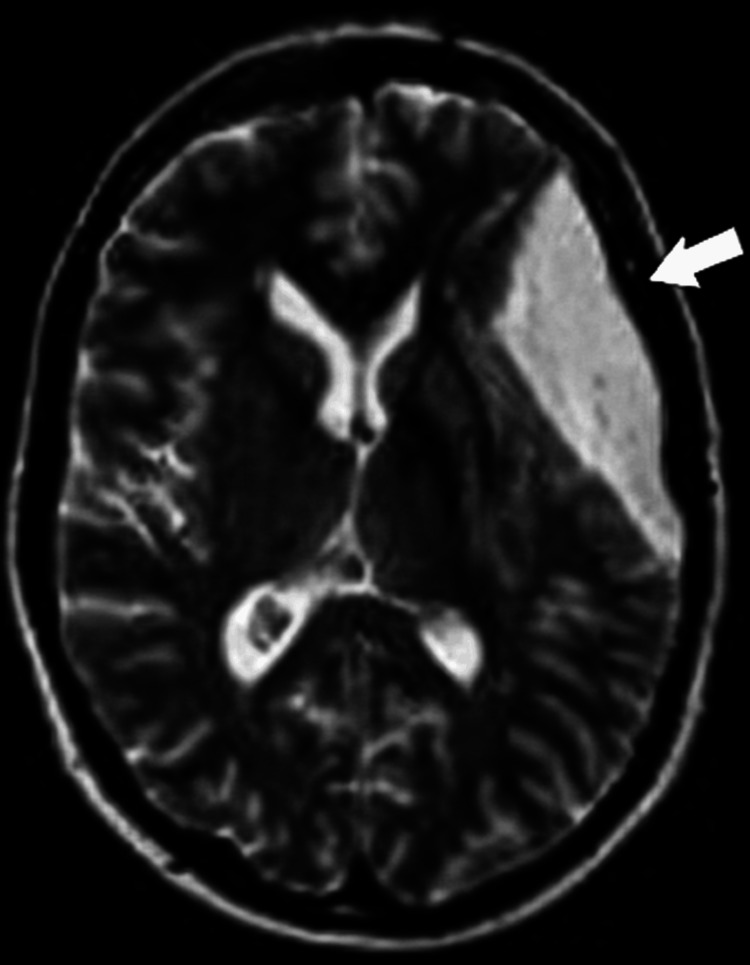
Axial T2-weighted brain MRI showing an incidental arachnoid cyst in the right anterior temporal region (arrow) with mild mass effect on adjacent brain parenchyma. MRI: magnetic resonance imaging
